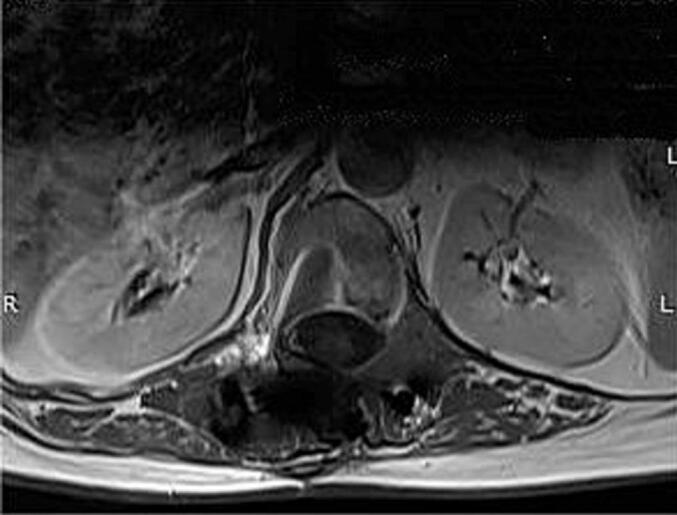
Postoperative thoracolumbar MRI. Axial T2-weighted image at the same level as Fig. 2 showing the complete resection of the cyst and the absence of repression of the left kidney which existed previously.
